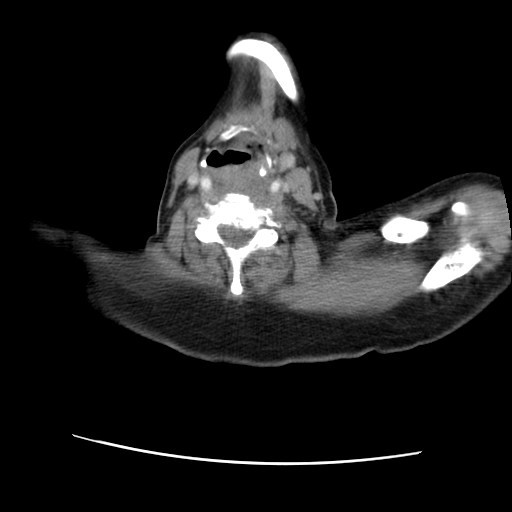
CT slice showing new lesion in oropharynx.
